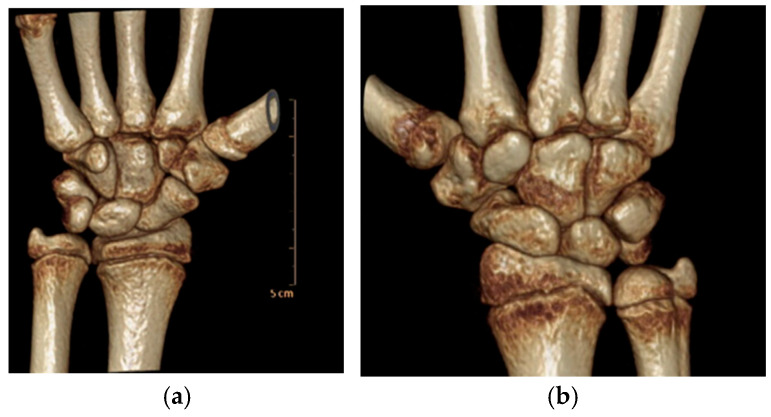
(a,b): CT was performed at two weeks (Figure 4), showing good joint congruency and no associated fractures.
